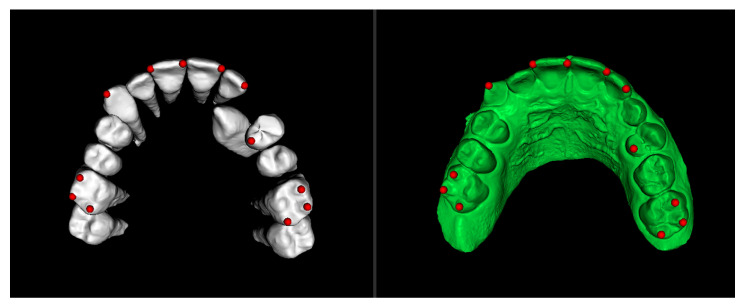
Superimposition of segmented teeth from initial CBCT and initial STL.
